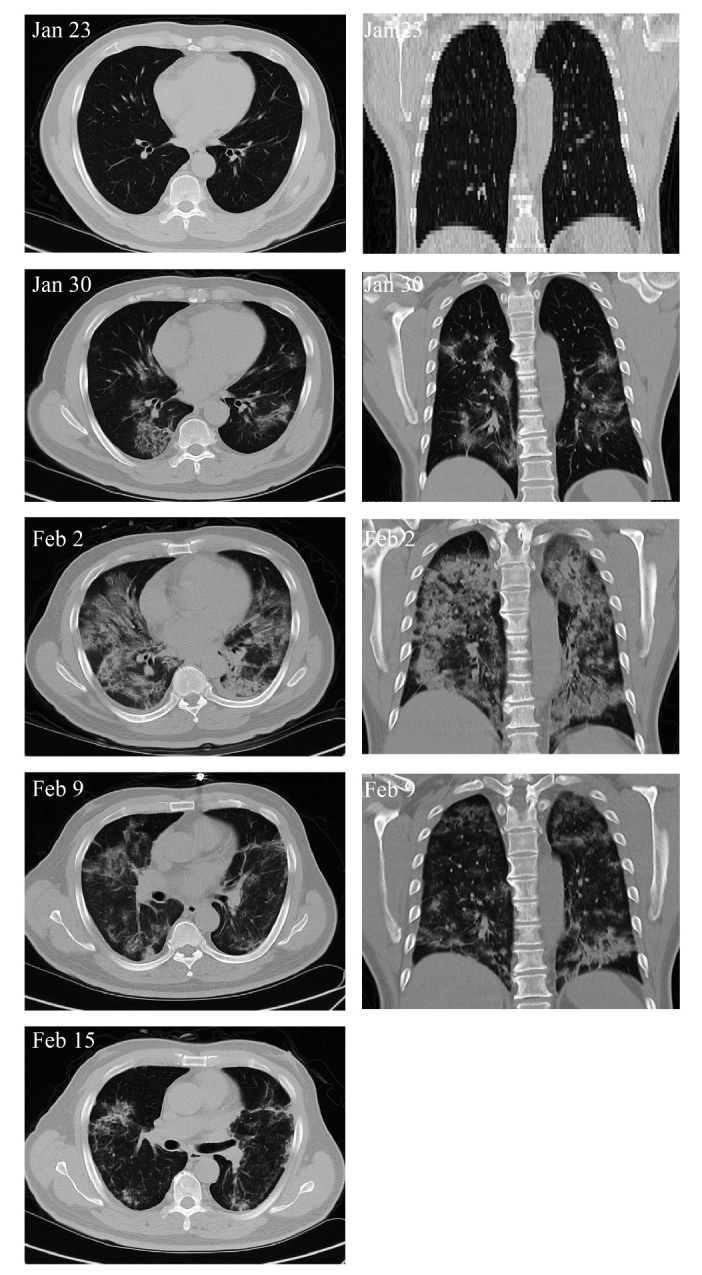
Chest computerized tomography (CT) images of the critically severe COVID-19 patient. On Jan 23, no pneumonia performance was observed. On Jan 30, ground-glass opacity and pneumonia infiltration occurred in multi-lobes of the double sides. Cell transplantation was performed on Jan 31. On Feb 2, the pneumonia invaded all through the whole lung. On Feb 9, the pneumonia infiltration faded away largely. On Feb 15, only little ground-glass opacity was residual locally.
